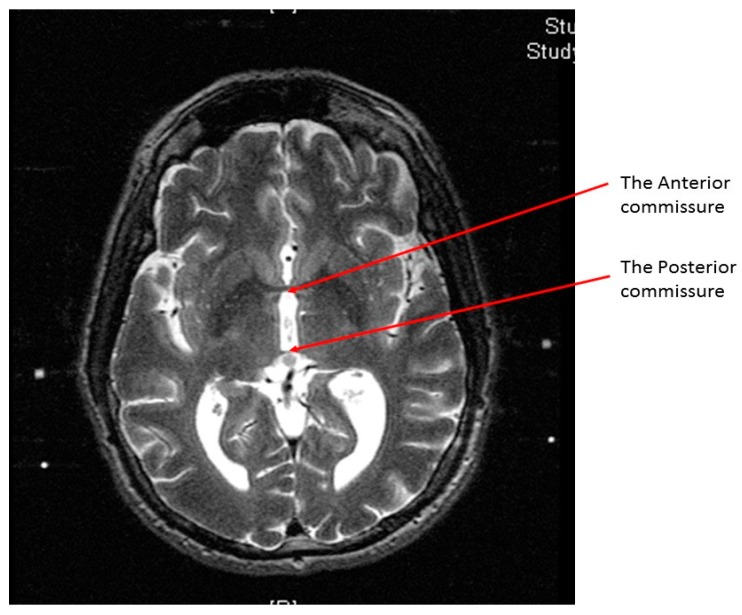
An axial T2 weighted magnetic resonance image showing the anterior commissure and the posterior commissure at the same cut.
